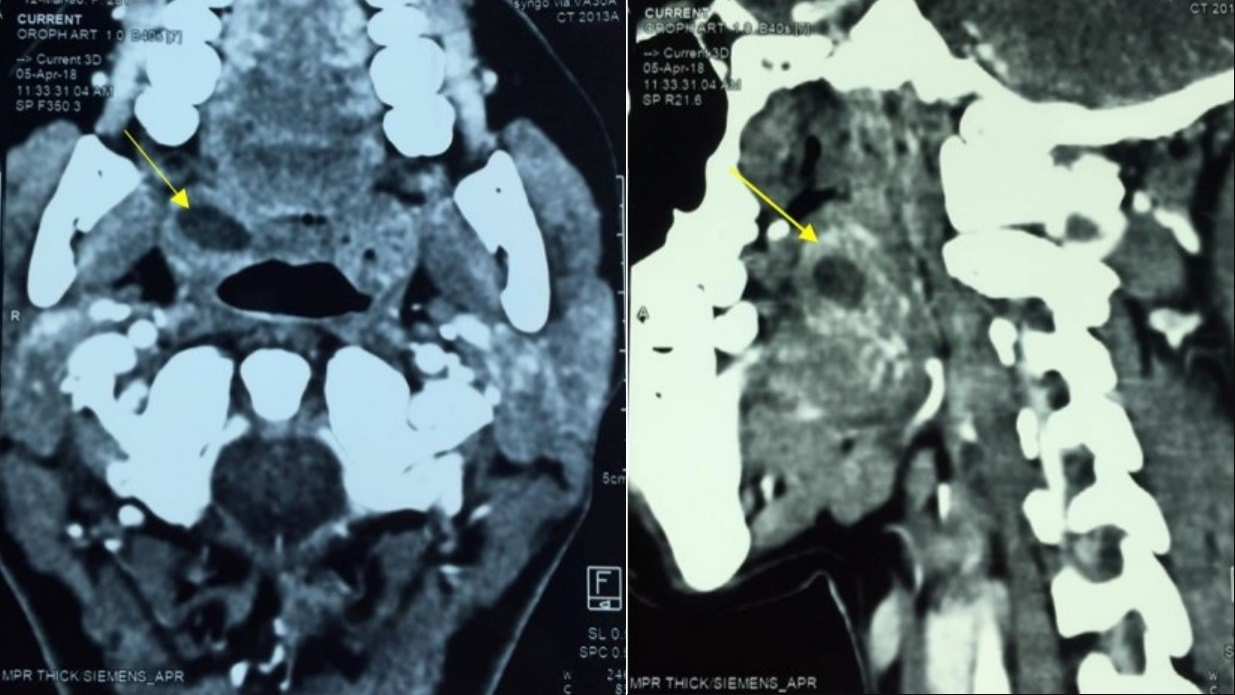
Axial injected computed tomography section of the oropharynx showed an hypo dense mass of the right palatine tonsil with peripheral enhancement
